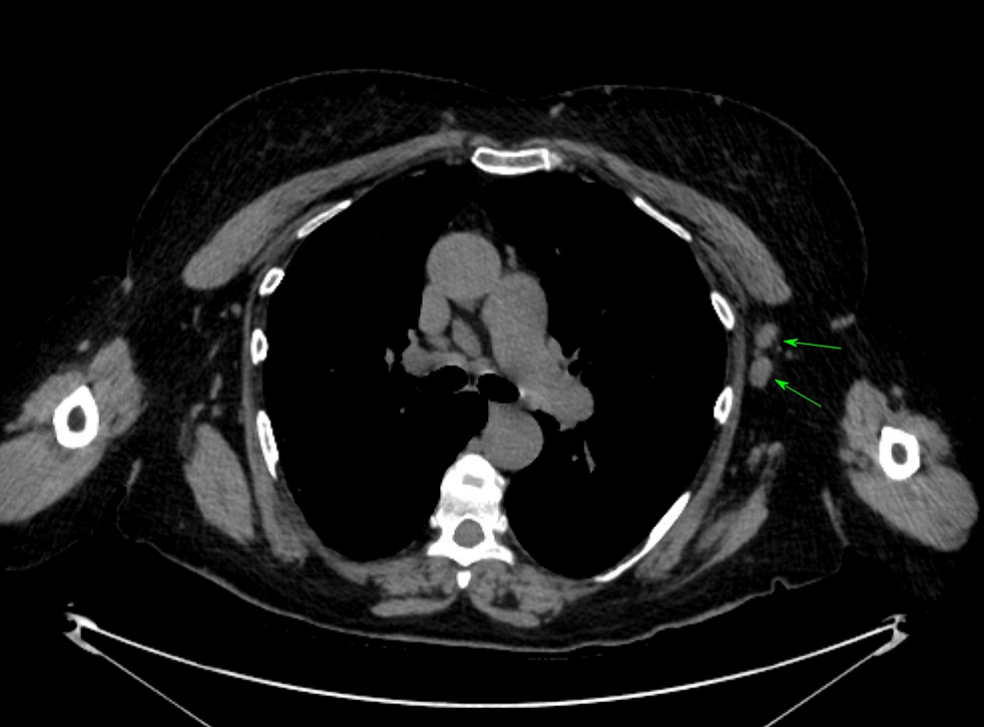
Axial view HRCT was performed for the evaluation of prominent broncho-vascular markings seen on a previous chest radiograph; it showed multiple enlarged left axillary lymph nodes (green arrows), and the patient was sent to the breast unit for triple assessment. HRCT: High-Resolution Computerized Tomography
